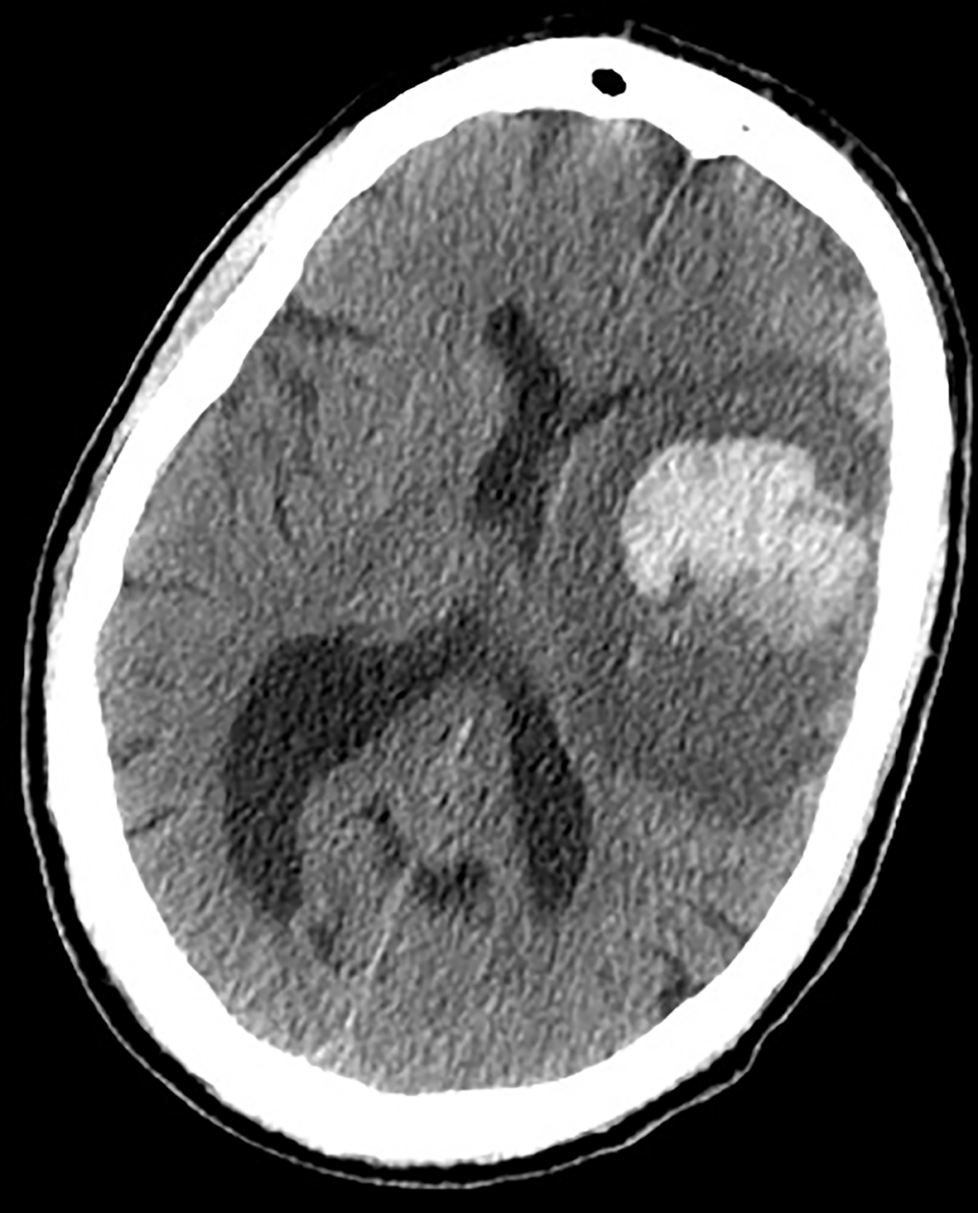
Earlier brain CT scan of a 71-year-old man presenting with acute onset of right hemiparesis: note the disproportionate hypodense edematous area surrounding the hematoma, not in accordance with the very acute phase of hemorrhage. An underlying unknown HGG was diagnosed.
